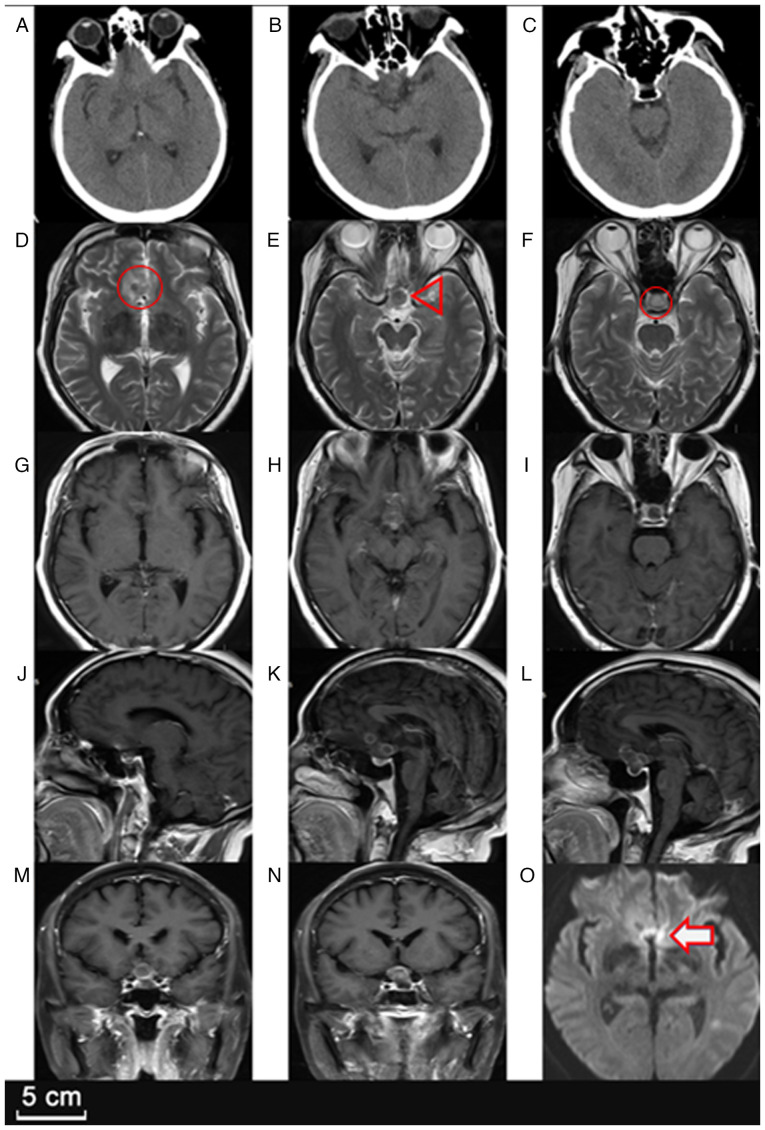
First imaging examination in Beijing Tiantan Hospital. (A-C) CT scans showing uneven sellar density. (D) Axial T2-weighted MRI showing irregular mixed signal intensity in the suprasellar and right frontal lobe areas (lesion indicated by a circle). (E) Axial T2-weighted MRI revealing that the lesion compresses the optic chiasma (indicated by an arrowhead), correlating with visual impairment. (F) Axial T2-weighted MRI showing mixed signals in the sellar region (indicated by a circle). (G-N) Axial, sagittal and coronal T1-weighted MRI images revealing annular enhancement of the saddle region and the right frontal lobe. (O) Axial diffusion-weighted MRI with a high signal intensity in the lesion area (indicated by an arrow), indicating limited diffusion in the area.
